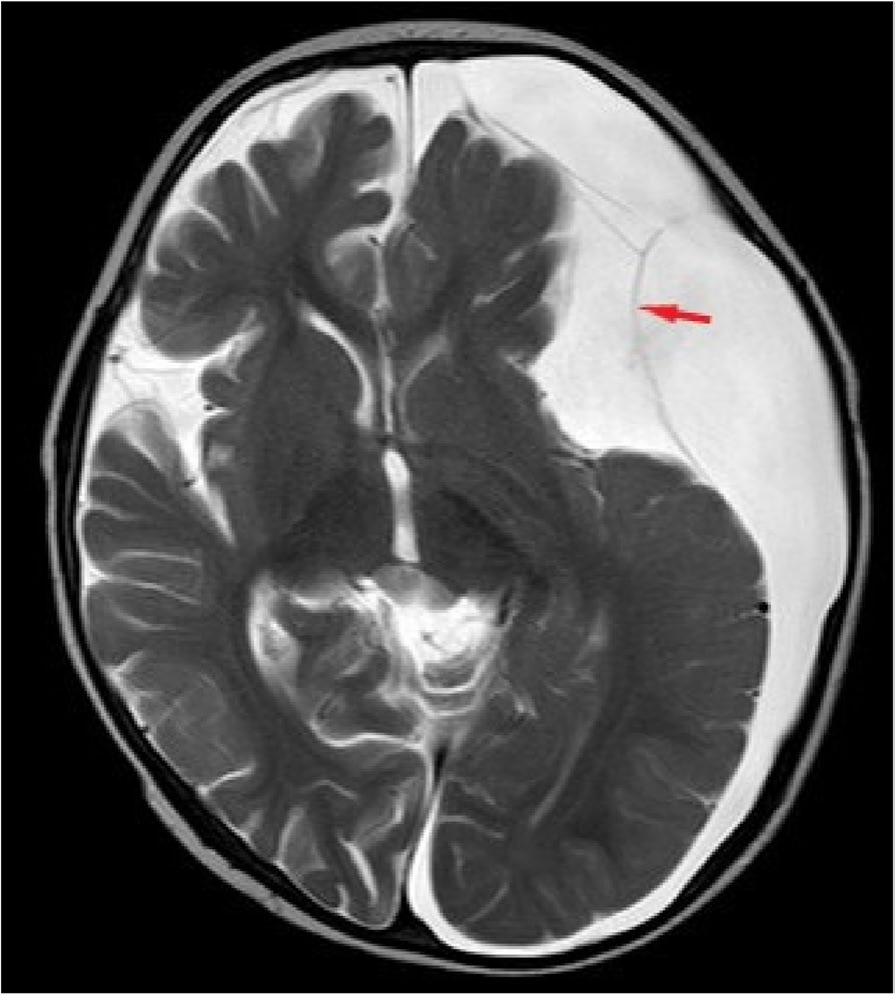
Cranial MRI demonstrating floating arachnoid signals (red arrow) within the subdural effusion, suggestive of a post-traumatic rupture of the left frontotemporal arachnoid cyst
